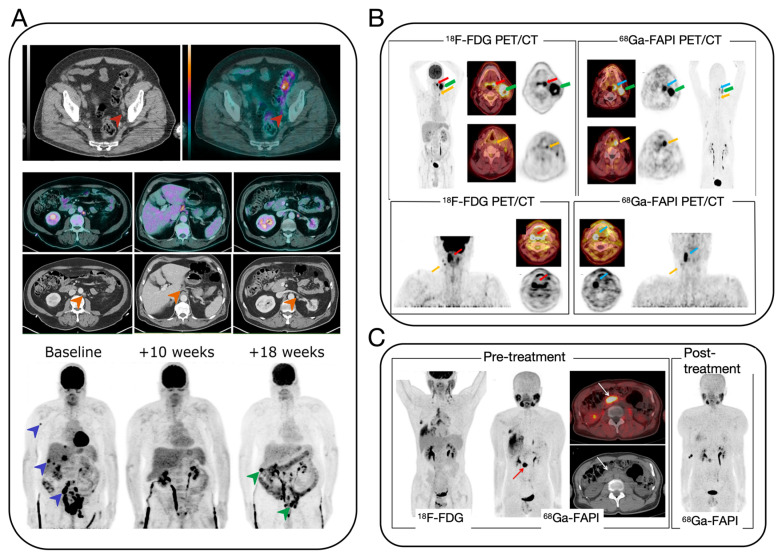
(A) ^18^F-FDG PET/CT images of three patients at different time points in the course of MIBC [19]. (B) PET/CT scans with the FDG and FAPI tracer of patient with palatine tonsil carcinoma and simultaneous hypopharyngeal carcinoma [23]. (C) Pre- and post-treatment PET/CT images of a 58-year-old man with IgG4-related disease [24].
